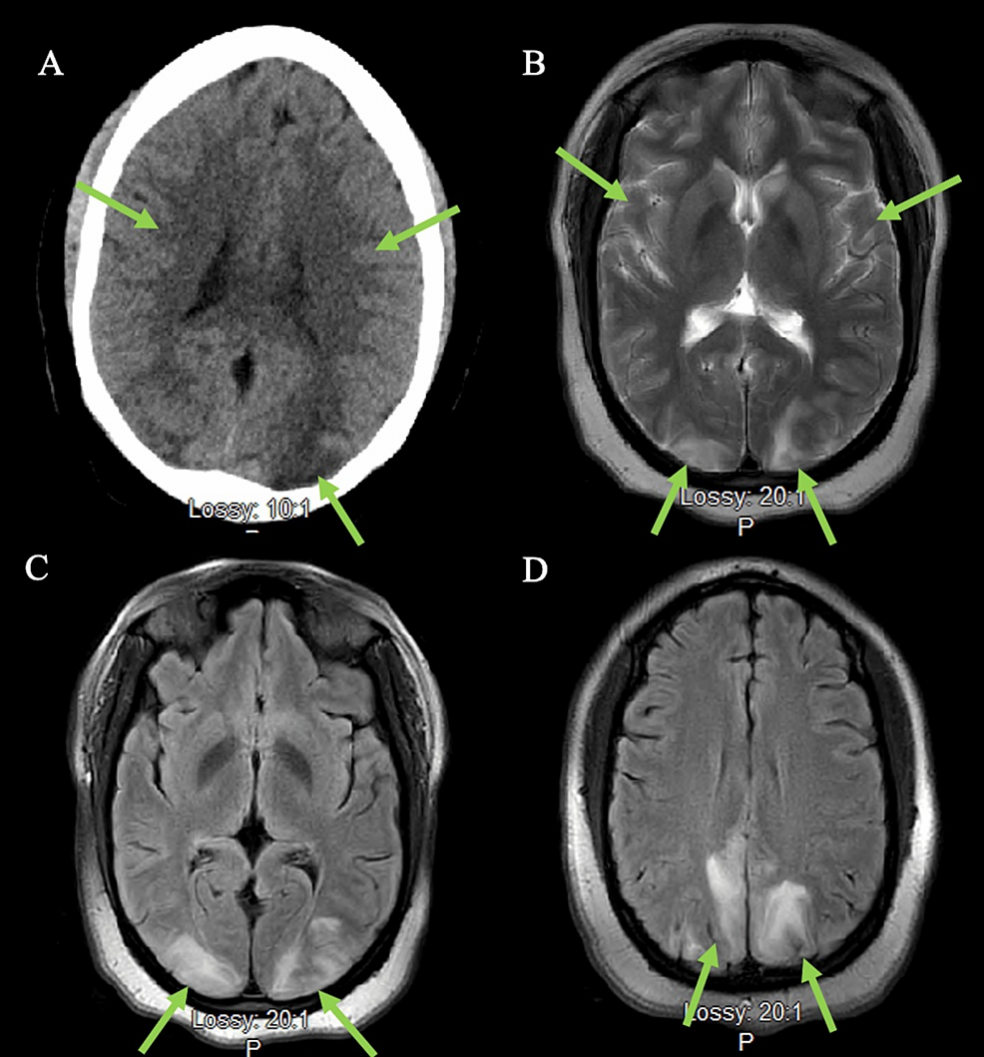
Neuroimaging findings A: Non-contrast head CT demonstrating vasogenic edema of the parieto-occipital lobes (see arrows). B: T2 MRI showing cortical and subcortical edema of bilateral parietal and occipital lobes (see arrows). C, D: Fluid attenuated inversion recovery (FLAIR) MRI demonstrating cortical edema centered on the occipital lobes and inferior margin of the parietal lobes (see arrows).
